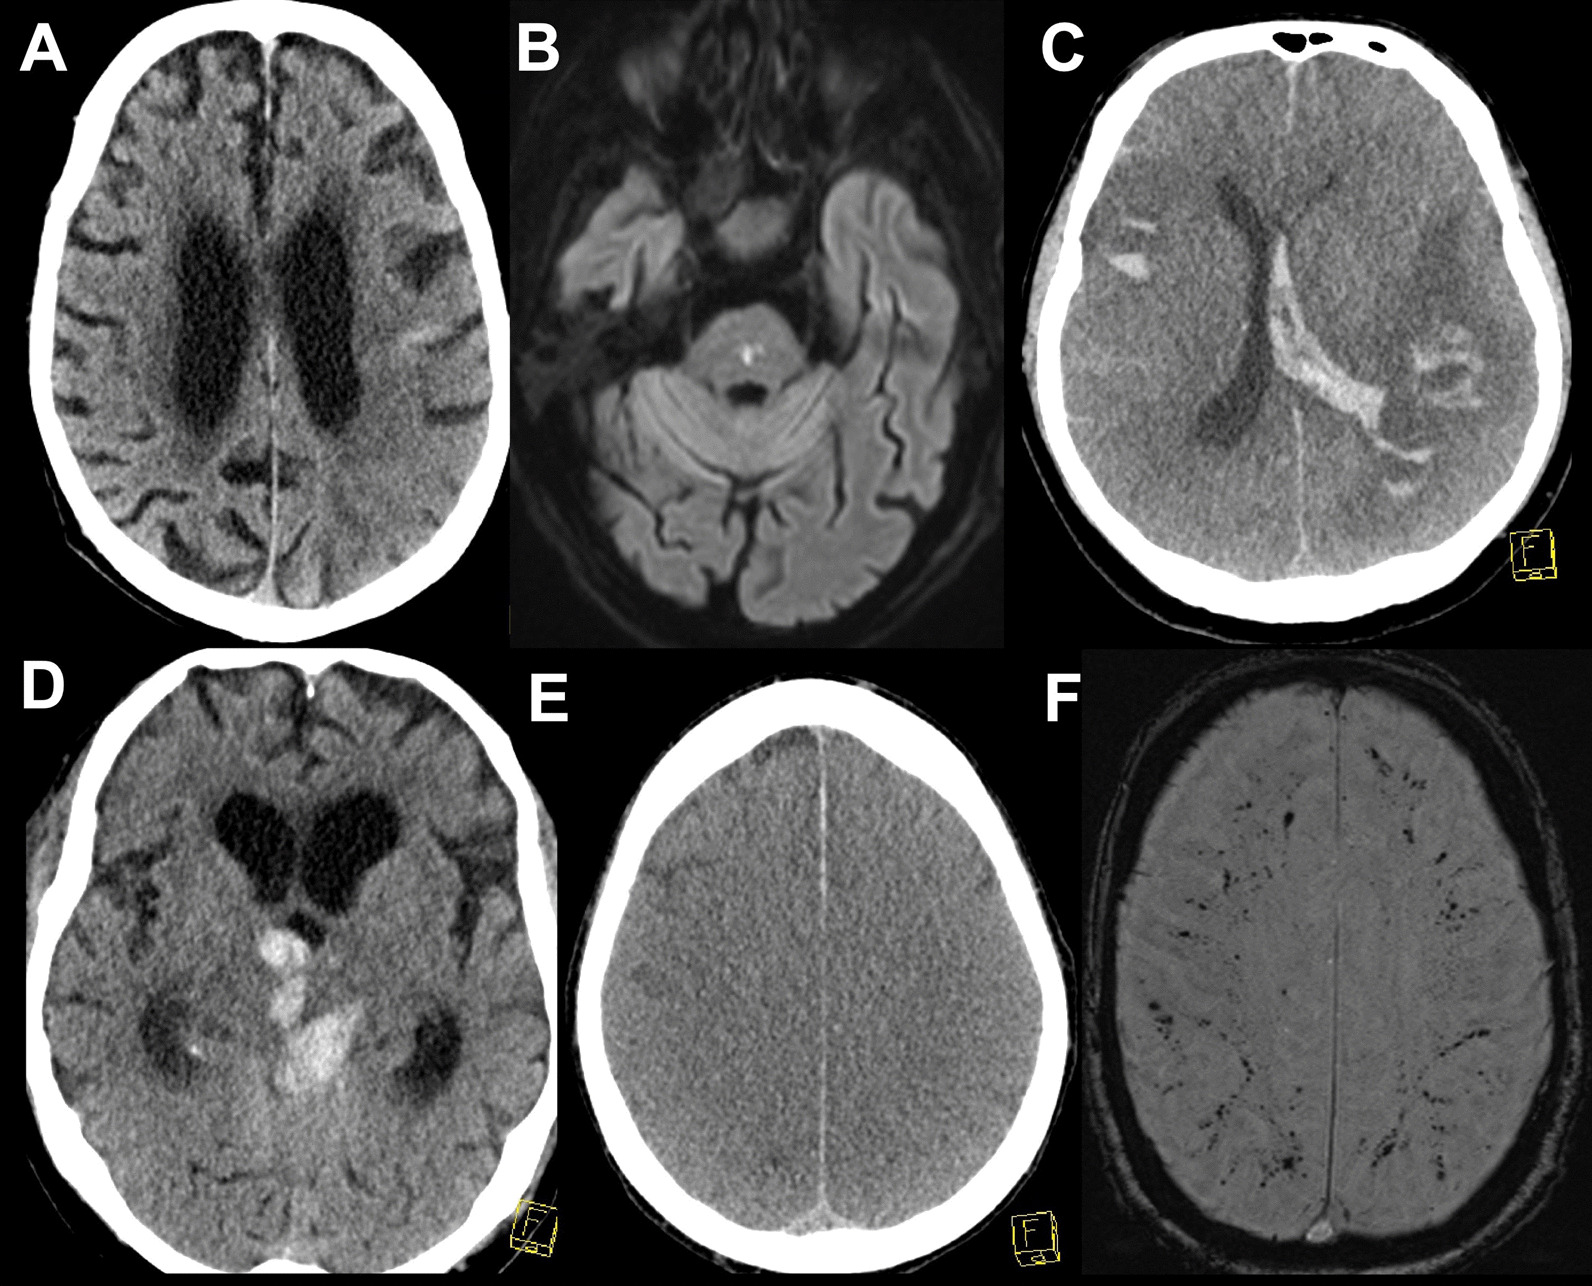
Characteristic imaging findings. A Computed tomography scan (CT) of a 90-year-old male patient with left parietal infarction. B Diffusion-weighted magnetic resonance imaging (DWI–MRI) of an 86-year-old male patient with a pontine infarction. C CT scan of a 67-year-old male patient with multiple intracerebral hemorrhages and intraventricular extension. D CT scan of a 78-year-old male patient with intracerebral hemorrhage with intraventricular extension. E CT scan of a 48-year-old female patient with severe hypoxia and generalized edema. F Susceptibility-weighted angiography MRI images of a 66-year-old male patient with multiple microbleeds
